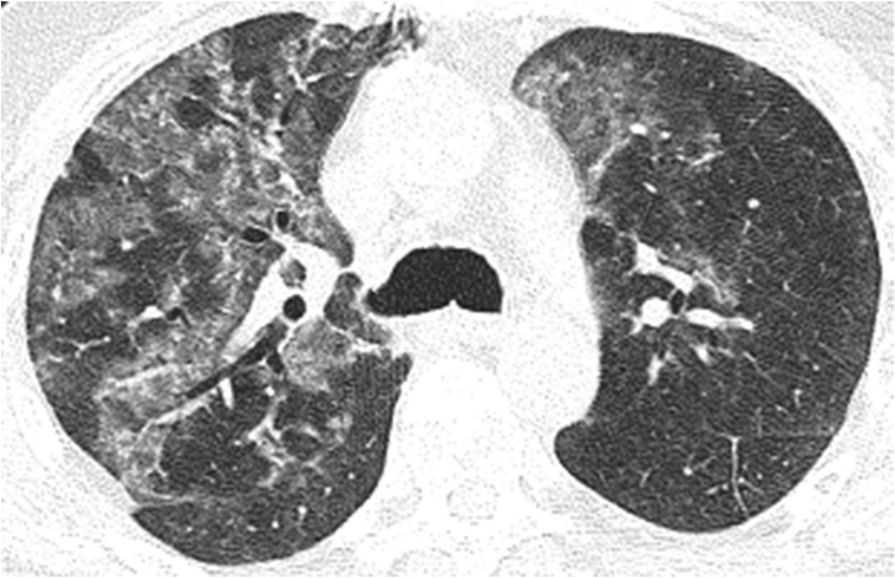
PJP/PCP in a 59-year-old male 3 years out from bilateral lung transplants for idiopathic pulmonary fibrosis. Axial CT image (lung window) at the level of the upper lobes shows right greater than left peribronchovascular distribution of ground glass opacities, which increased on subsequent imaging during that admission (not shown). The patient was originally admitted with fever and constitutional symptoms with suspicion for post-transplant lymphoproliferative disorder, however diagnosis of PJP/PCP was consistent based on the imaging, history of non-adherence to prophylaxis for approximately one year, and sputum PCR results
